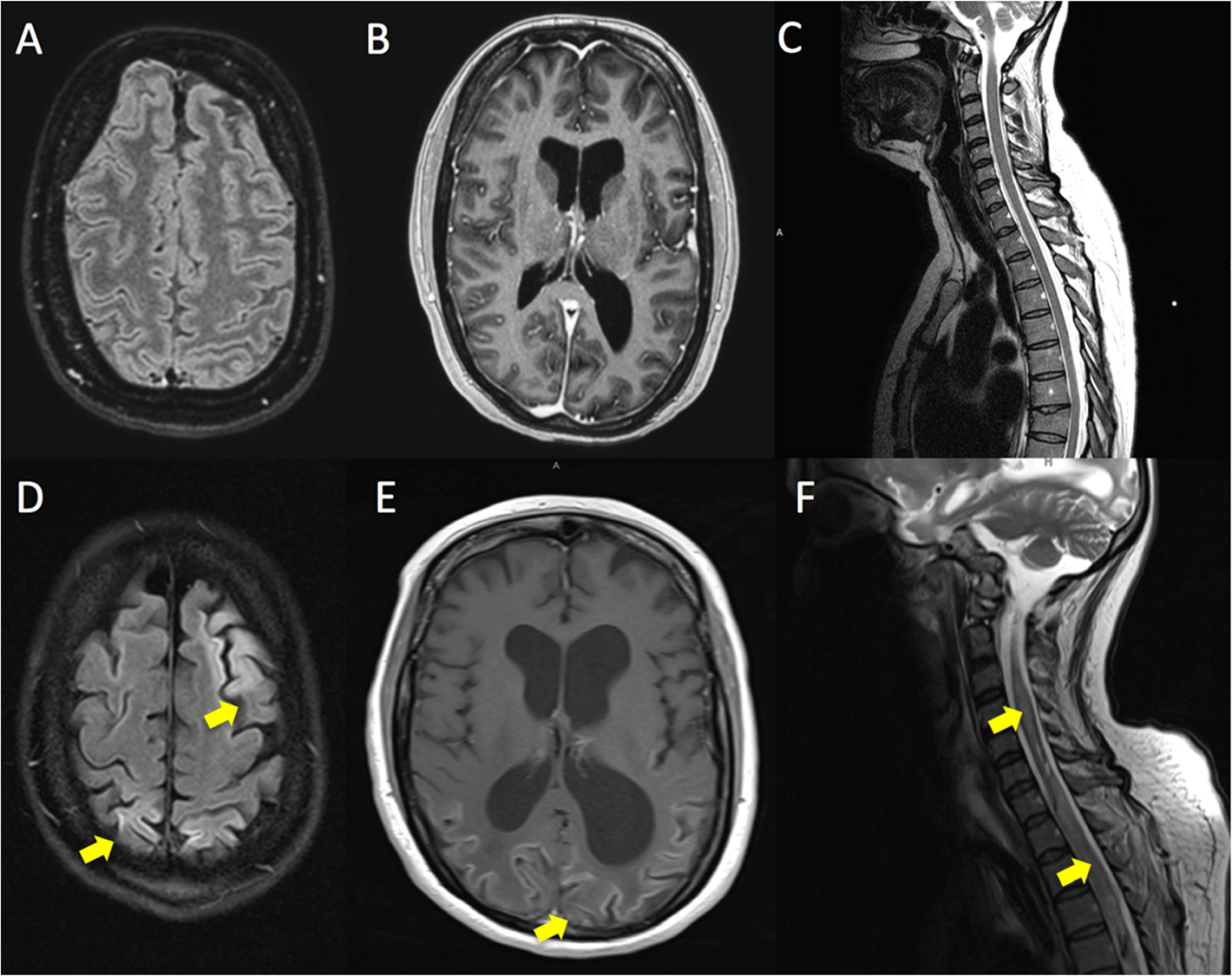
Axial MRI. MRI findings from initial presentation (A-C, D-F). MRI findings of FLAIR (A, D), T1-weighted image with Gd enhancement (B, E) and T2w weighted spinal MRI (C, F). Initial MRI of the brain (A, B) revealed subtle signs of meningitis, MRI of the spinal cord (C) showed no pathological findings. MRI of the brain after admission to ICU (D, E) revealed cortical and subcortical lesions bihemispherically with parieto-occipital emphasis as well as hyperintense signal alterations of the mesial temporal lobes. MRI of the spinal cord (F) revealed expanded oedema of medulla oblongata. (Marked with yellow arrows)
